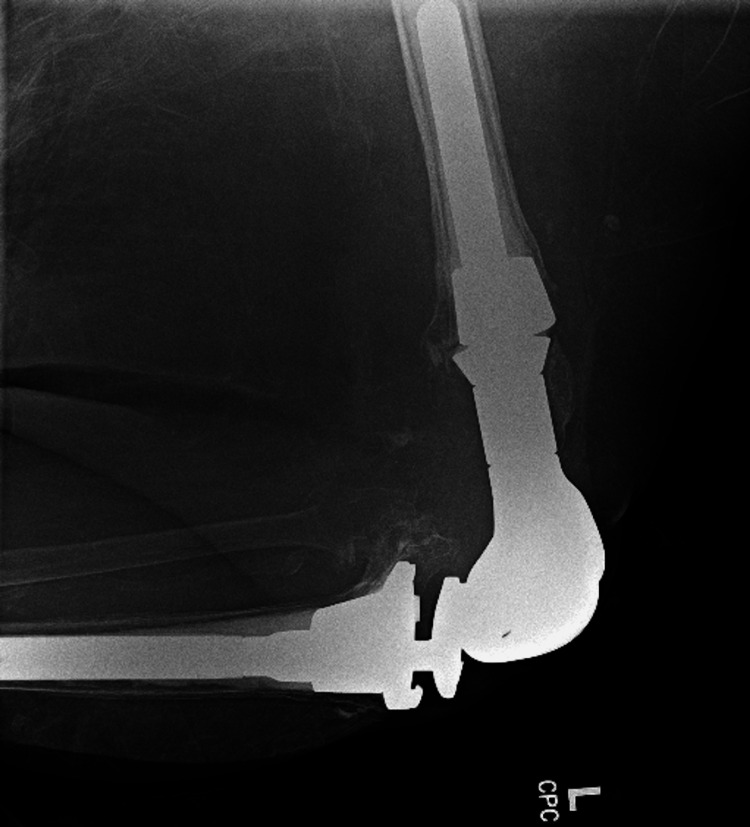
Left knee X-ray This X-ray highlights the components of the knee joint. The hinged knee joint prosthesis that inserts into the femur. A long stem prosthesis is seen in the tibia.
